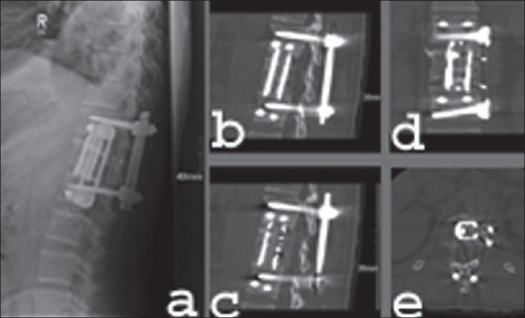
Post operative x-ray lateral view (a) reconstructed sagittal CT scan (b and c), coronal reconstruction (d) and axial CT scan (e) after thoracoscopic anterior decompression of the same patient shows bi-segmental reconstruction with distractable cage filed with cancellous bone and reinforced with ante-rior instrumentation
